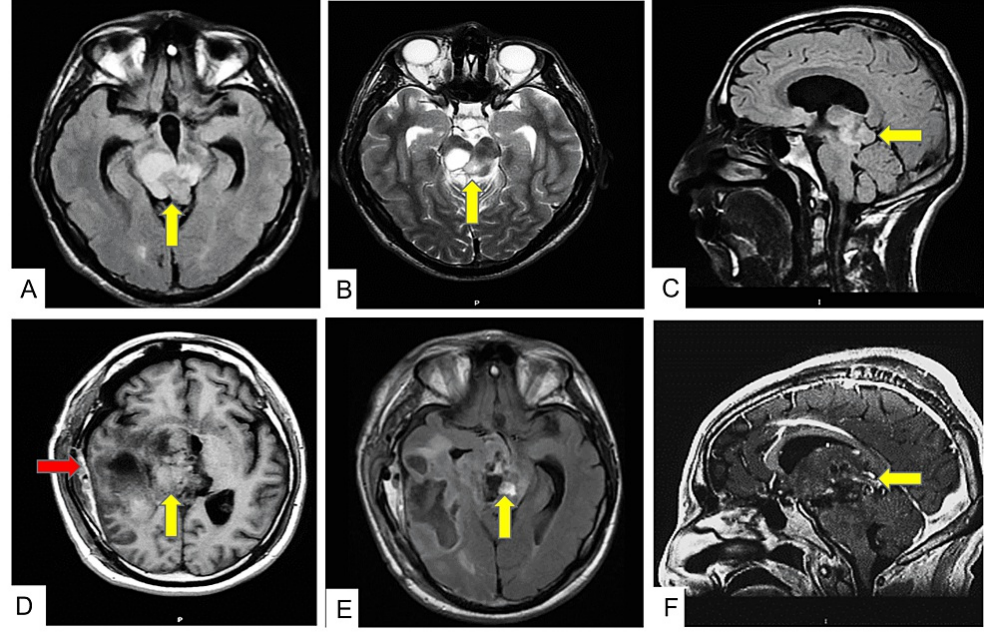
Brain magnetic resonance imaging. First MRI with pineal region tumor. (A) Hyperintense signal in the pineal region in axial T2-FLAIR (yellow arrow). (B) Multiloculated in axial-T2 (yellow arrow). (C) Sagittal-T2-FLAIR (yellow arrow). (D) Second MRI with post-surgical changes (red arrow), tumor growth in axial T1 (yellow arrow). (E) Hyperintense signal in axial T2-FLAIR (yellow arrow). (F) Sagittal T1 with gadolinium enhancement (yellow arrow). MRI: magnetic resonance imaging; FLAIR: fluid-attenuated inversion recovery
